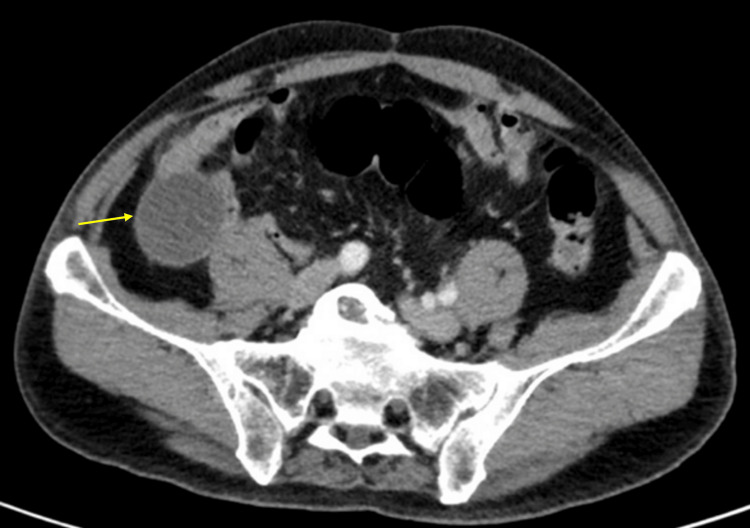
Axial section of computed tomography imaging showing appendiceal mucocele (arrow).
